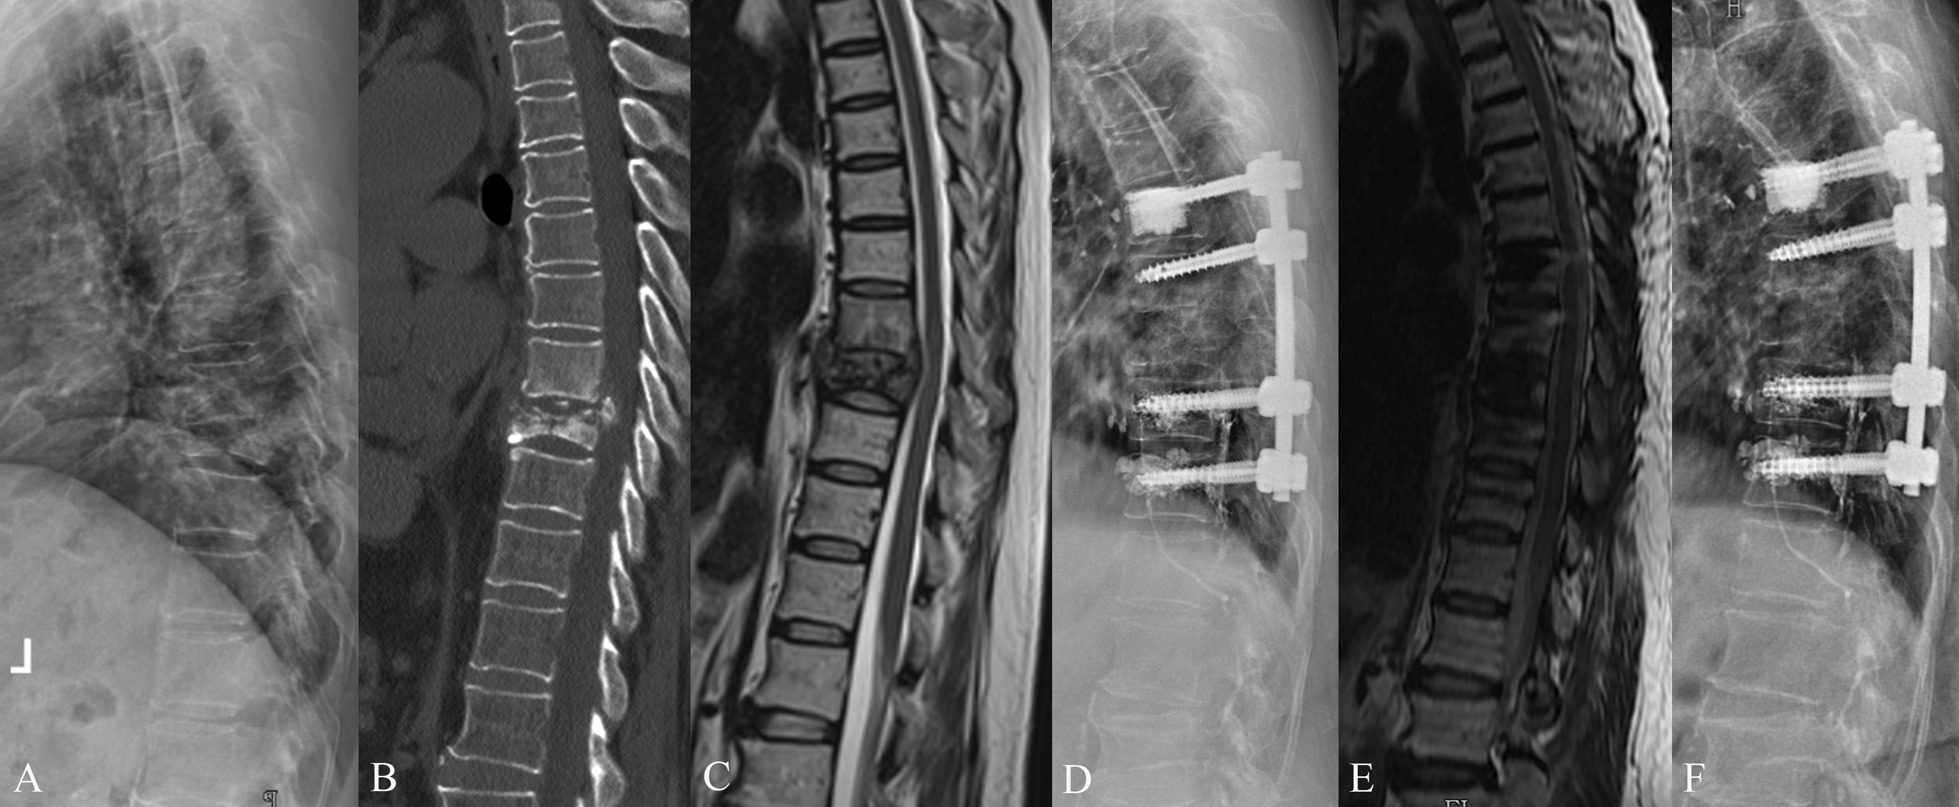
A 71-year-old male with T9-10 tuberculosis and severe osteoporosis. The patient underwent one stage posterior debridement, bone grafting, and fixation with cement-augmented pedicle screws. A–C Preoperative lateral X-ray, sagittal CT scan, and sagittal MRI scan images demonstrated bone destruction and sequestra. D Postoperative lateral X-ray image indicated cement leakage in the spinal canal and paravertebral vessel. E Sagittal MRI scan at 6-month follow-up. F X-ray image at final follow-up
